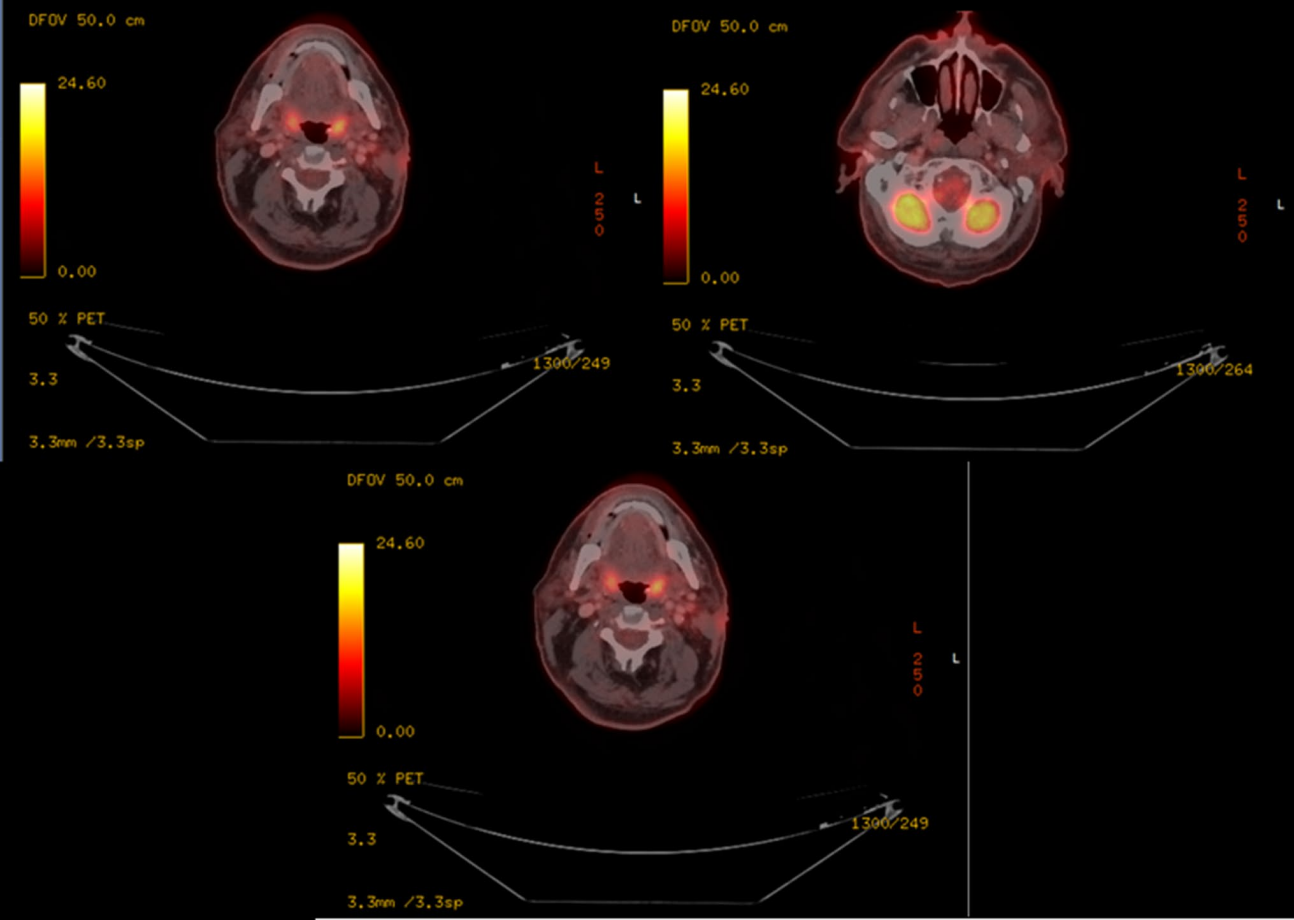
A complete metabolic response already 14 weeks after immunotherapy of Pembrolizumab initiation despite remaining morphological masses on CT
